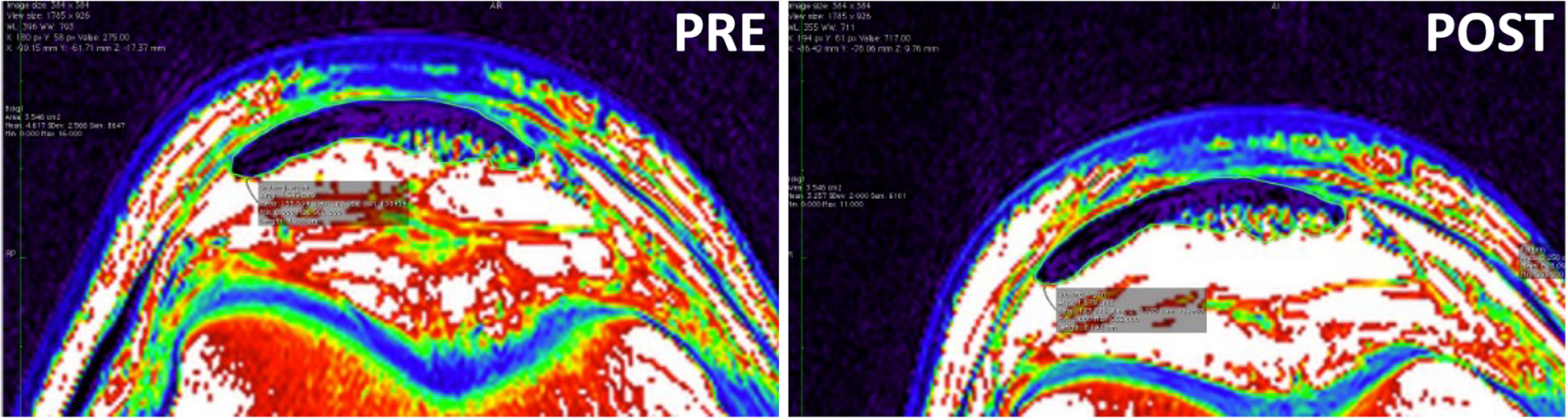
Typical magnetic resonance images of the proximal patellar tendon from the same subject before (PRE) and after (POST) 12 months intervention. The two images are obtained at the same location
